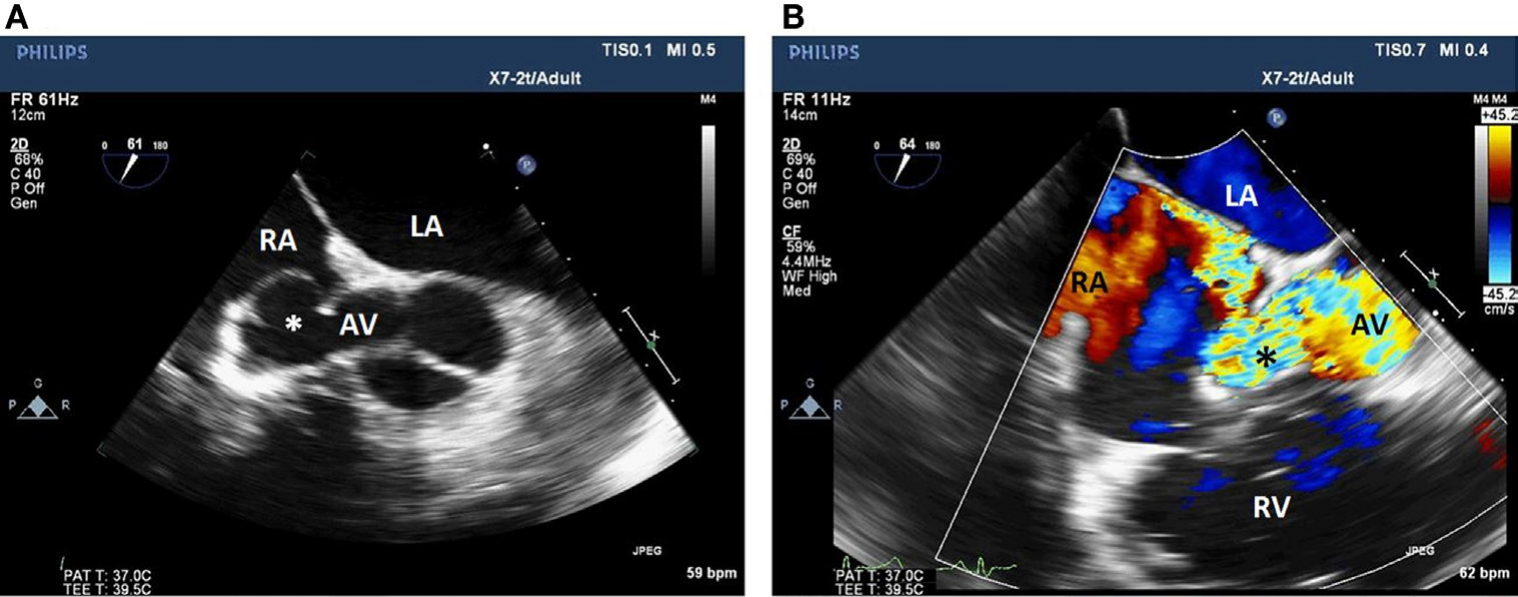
Two-dimensional echocardiogram short-axis view of the aortic valve (A) with color flow Doppler (B) showing a fistula between the non-coronary sinus of Valsalva aneurysm and the RA. LA, left atrium; RA, right atrium; AV, aortic valve; Asterix, sinus of Valsalva aneurysm; RV, right ventricle.
